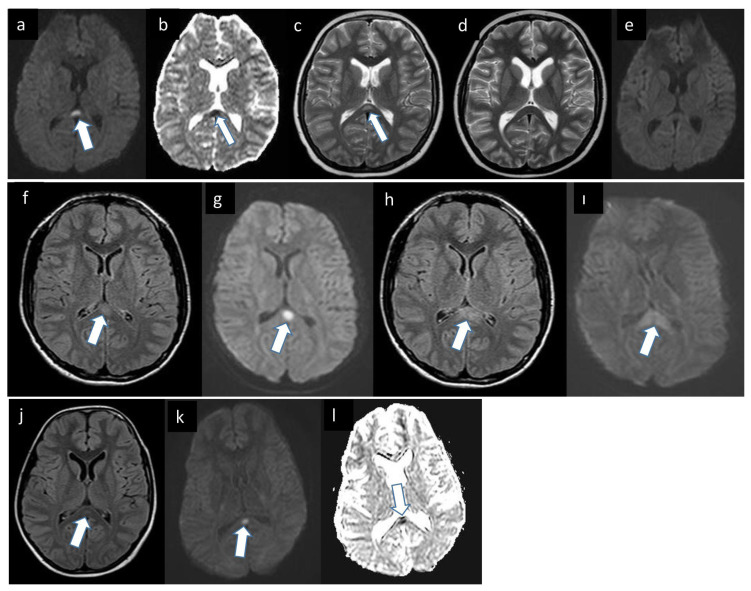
(a–l) legend: a: Diffusion-weighted image of the patient shows restricted diffusion in the splenium of the corpus callosum *(arrow)*. b: Note the low ADC value as a dark signal *(arrow)* in the ADC map. c: T2 weighted image demonstrates high signal lesion in the splenium of the corpus callosum compatible with edema *(arrow)*. d,e: On follow-up MRI, the lesion in the splenium of corpus callosum was totally gone. f: FLAIR image of Brain MRI shows high signal lesion in the splenium of the corpus callosum compatible with edema *(arrow)*. g: Diffusion-weighted image of the patient shows restricted diffusion in the splenium of the corpus callosum compatible with cytotoxic edema *(arrow)*. h,ı: On the follow-up MRI, the FLAIR and DWI images reveal the enlargement of edema in the splenium of corpus callosum (arrows). j: FLAIR image of the patient demonstrates high signal lesion in the splenium of the corpus callosum*(arrow)*. k: Diffusion-weighted image shows restricted diffusion in the splenium of the corpus callosum *(arrow)*. l: Note the low ADC values as a dark signal *(arrow)* in the ADC map regarding cytotoxic edema.
